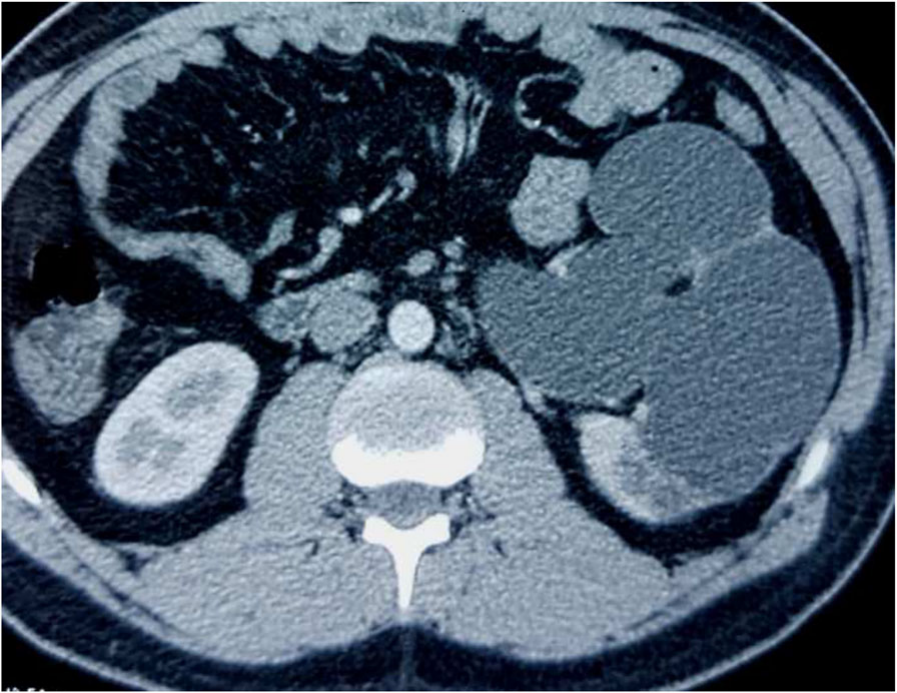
Axial enhanced computed tomography urography showing severe left hydronephrosis
